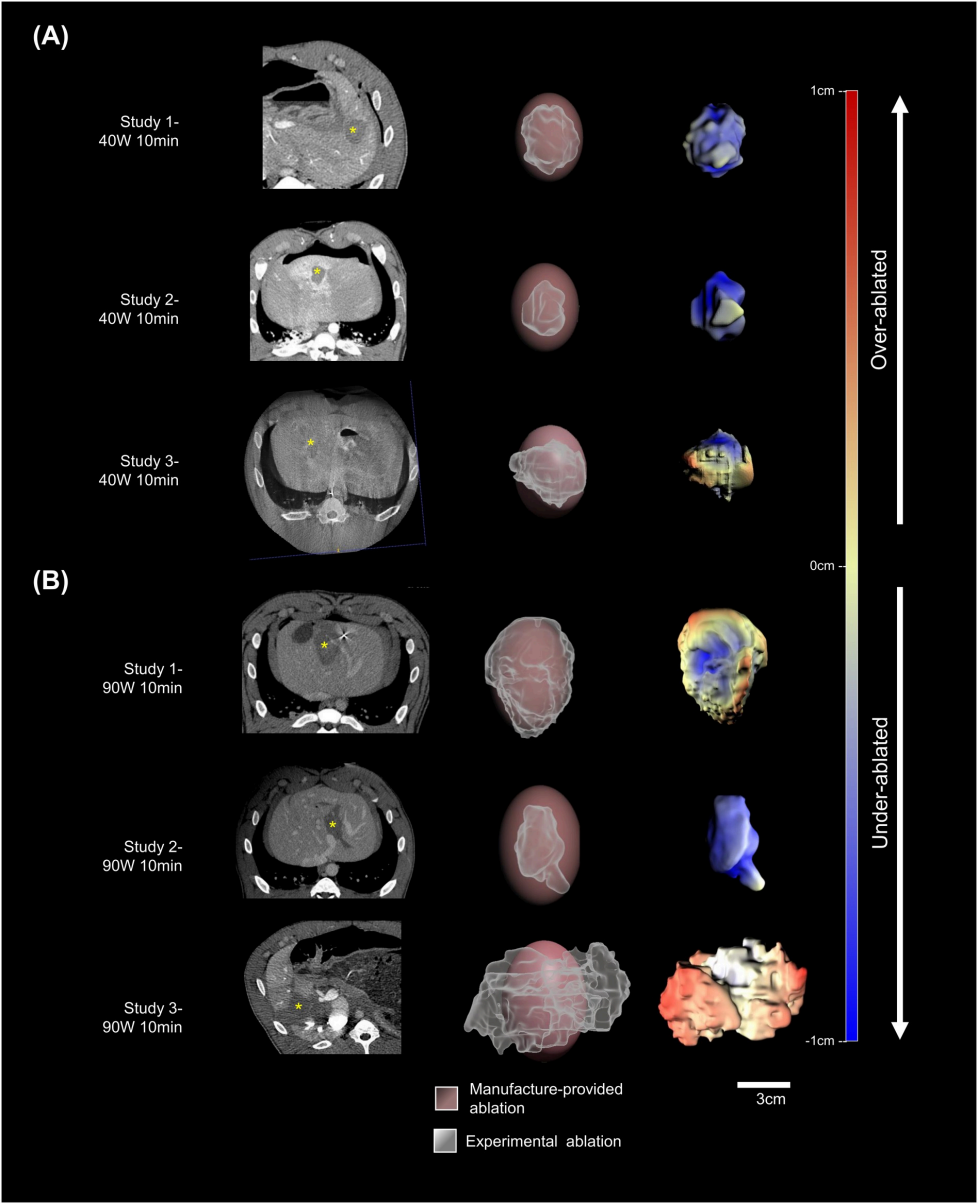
Characterization of microwave ablation zones *in vivo*. The results of (A) 40W and (B) 90W 10-minute microwave ablations *in vivo* are shown (n = 3 each). Portal phase contrast-enhanced CT (left column) after ablation demonstrating the ablation zone (*). Registered overlay of surface renderings of the manufacturer-provided (pink) and experimental segmented (white) ablation zones (middle column). The quantified differences in distance between expected and actual ablation zone surfaces are represented in the color-coded scale on the surface rendering of the ablation zone (right column). “Under-ablated” refers to a surface of the ablation zone that was inside the expected ablation zone while “over-ablated” refers to the surface of the ablation zone lying outside of the expected ablation.
